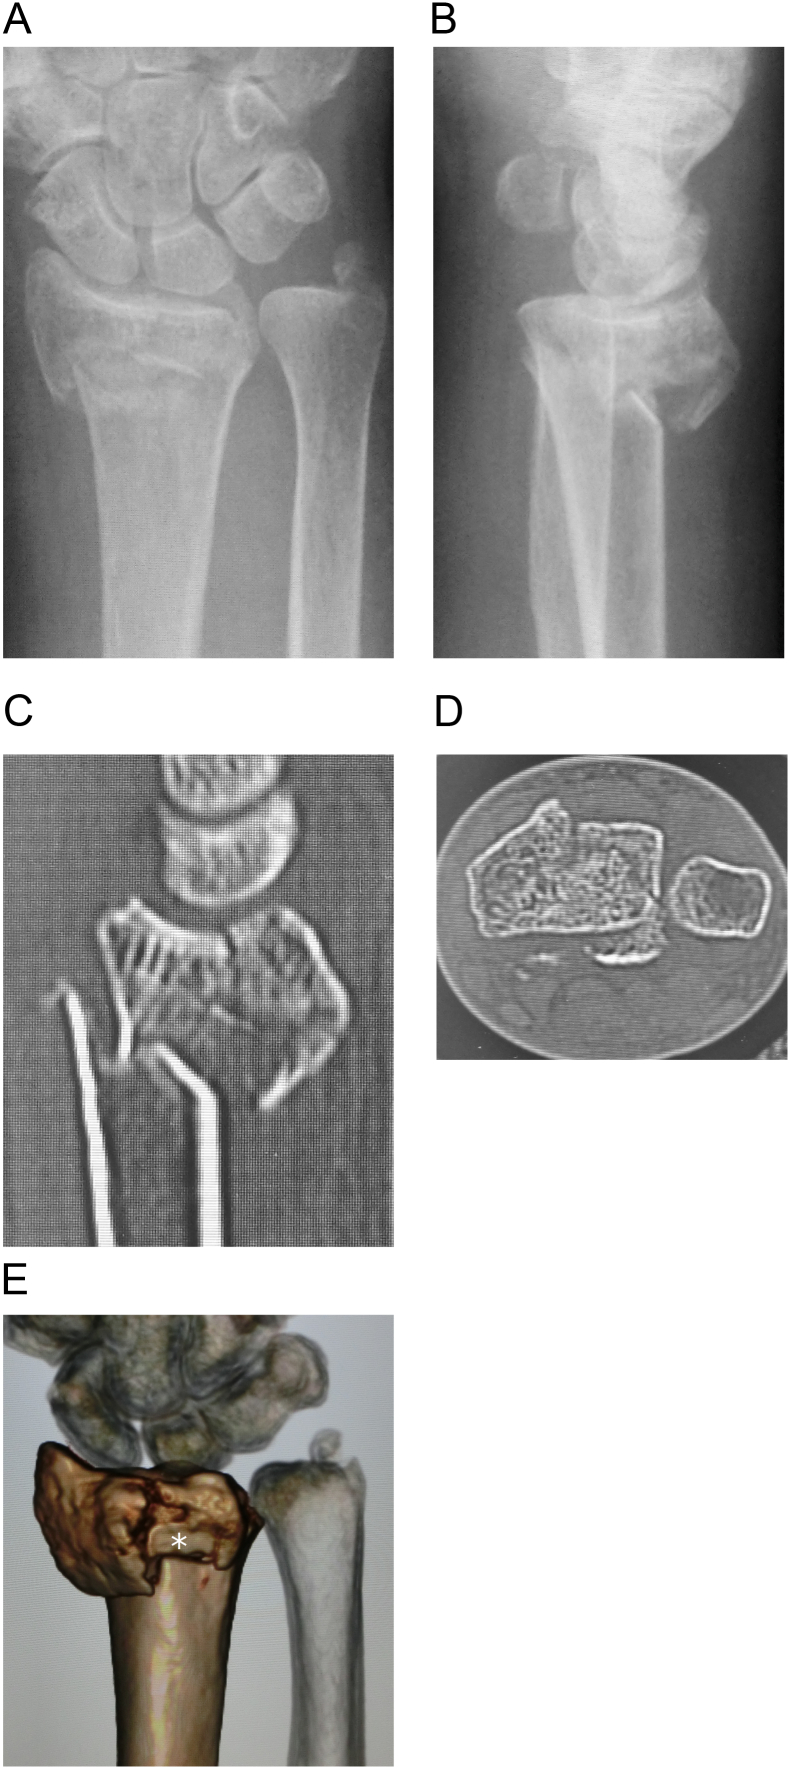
Preoperative image showing the comminuted intra-articular distal radial fracture. A and B: preoperative radiographs, C and D: sagittal and axial views of computed tomography images, E: three-dimensional computed tomography image. The dorsomedial lunate facet fragment and the dorsal roof fragment (white asterisk) are visible.
